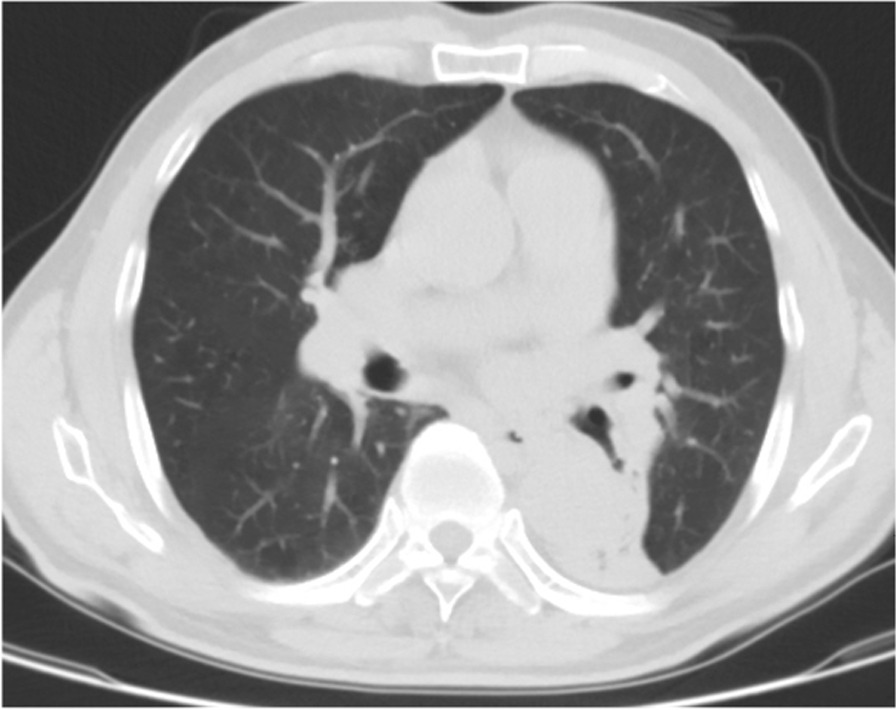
Chest computed tomography plain scan: left lower lobe bronchus partially occluded, left lower lobe consolidation
